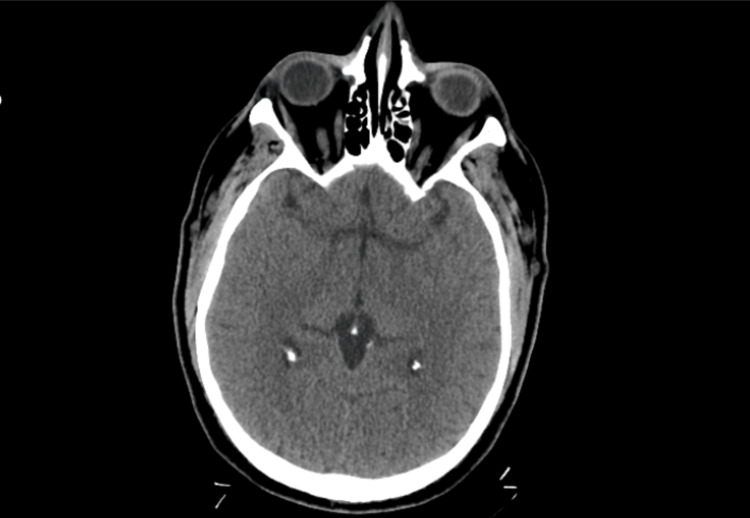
Non-contrast CT of the head showed no intracranial hemorrhage, infarct, or mass.
